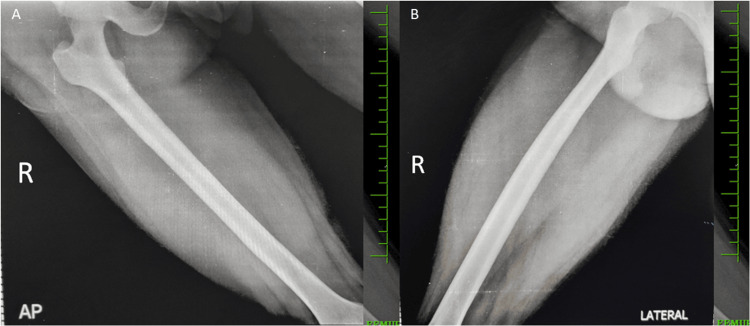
Radiograph of the right thigh: (A) anteroposterior and (B) lateral projection revealed no obvious abnormality.
